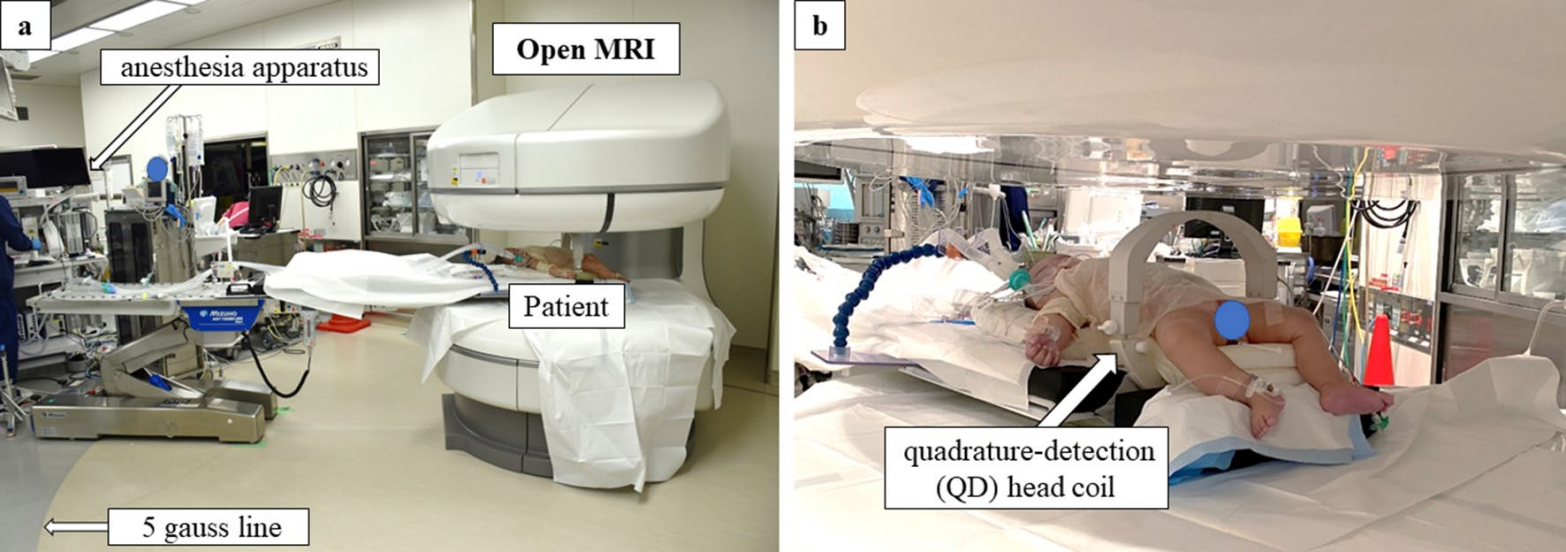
a Long respiratory tube between the anesthetic instrument and the gantry was needed for general anesthesia. b Patient was inserted into the gantry for open MRI scanning. The quadrature-detection (QD) head coil was attached around the patient’s pelvis
